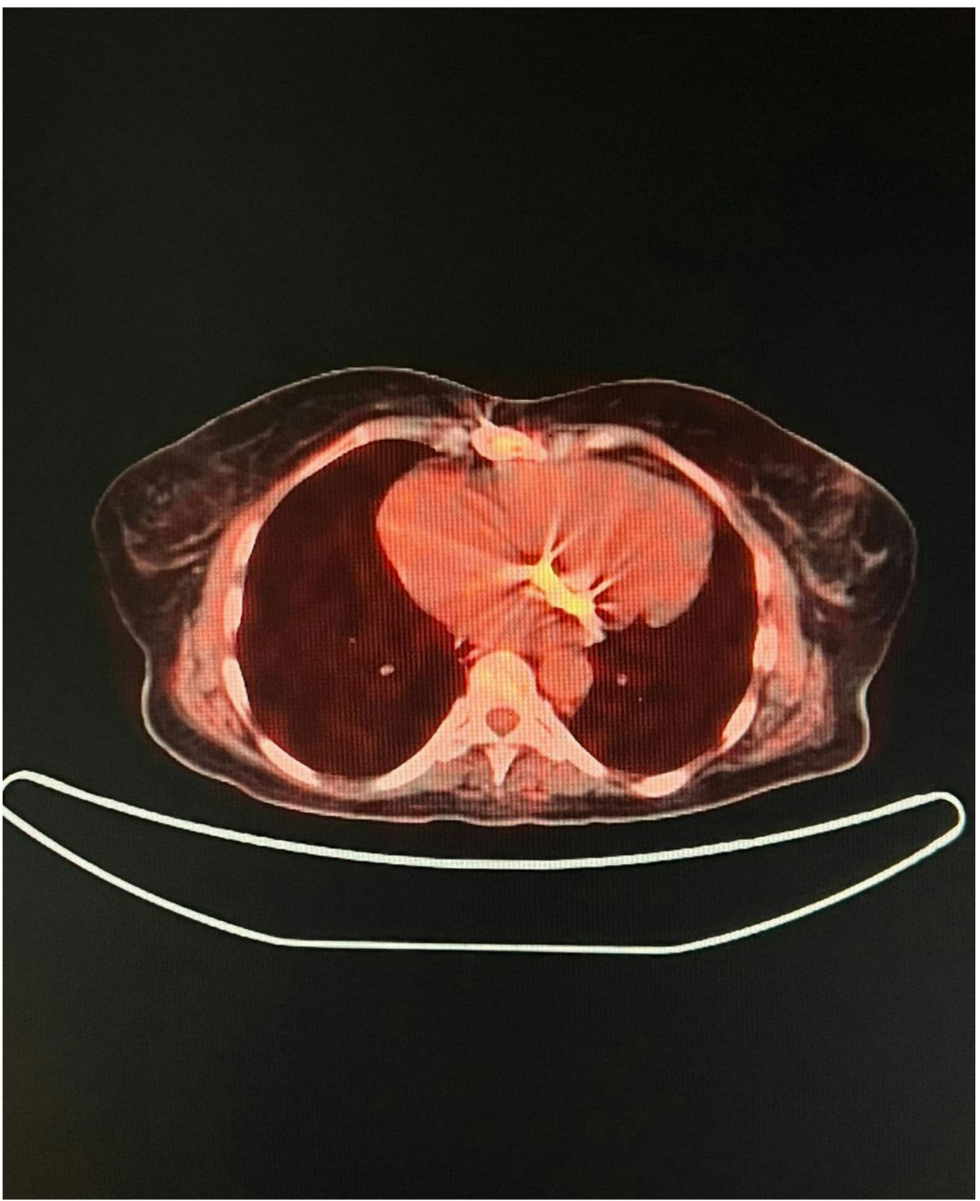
Positron emission tomography (PET) scan demonstrating pathological fluorodeoxyglucose (FDG) uptake at the site of the mitral valve prosthesis, consistent with active inflammatory or infectious process
